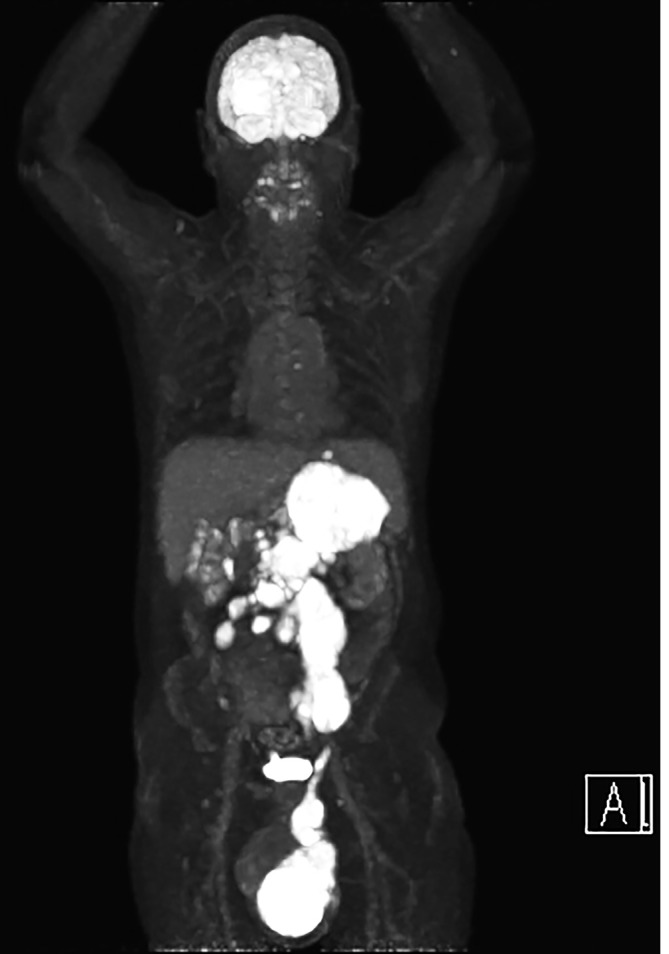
3D reconstruction following the FDG-PET scan which demonstrates the extent of the tumour spread.
